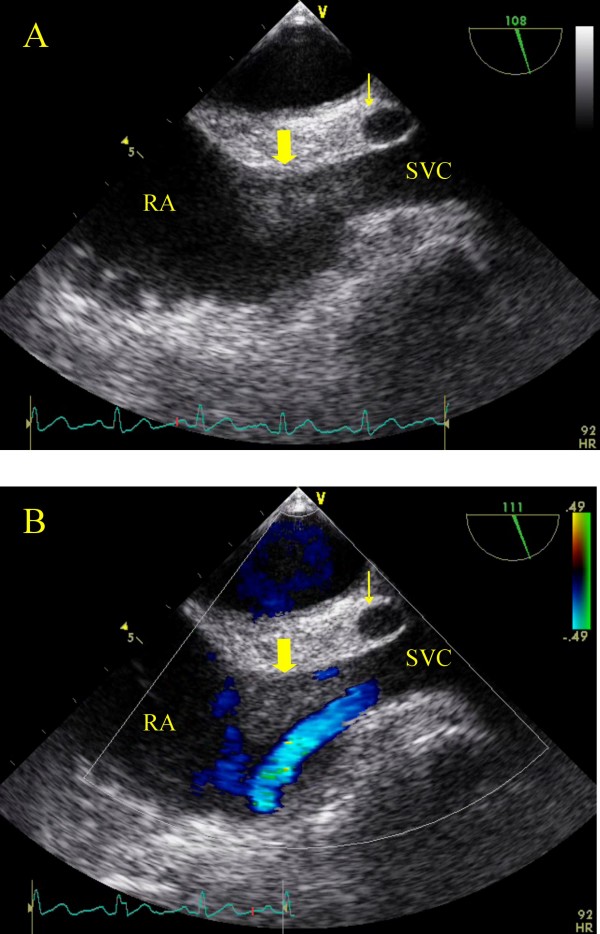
Transesophageal echocardiographic imaging-bicaval view-confirmed a 2.5 × 1.5 round solitary lesion with distinctive borders attached in the SVC, protruding into the right atrium (Thick arrow). The echo lucent area-marked with the thin arrow-most probably presents an abscess of the interatrial septum (A, B). Flow from the superior vena cava was obstructed (B).
